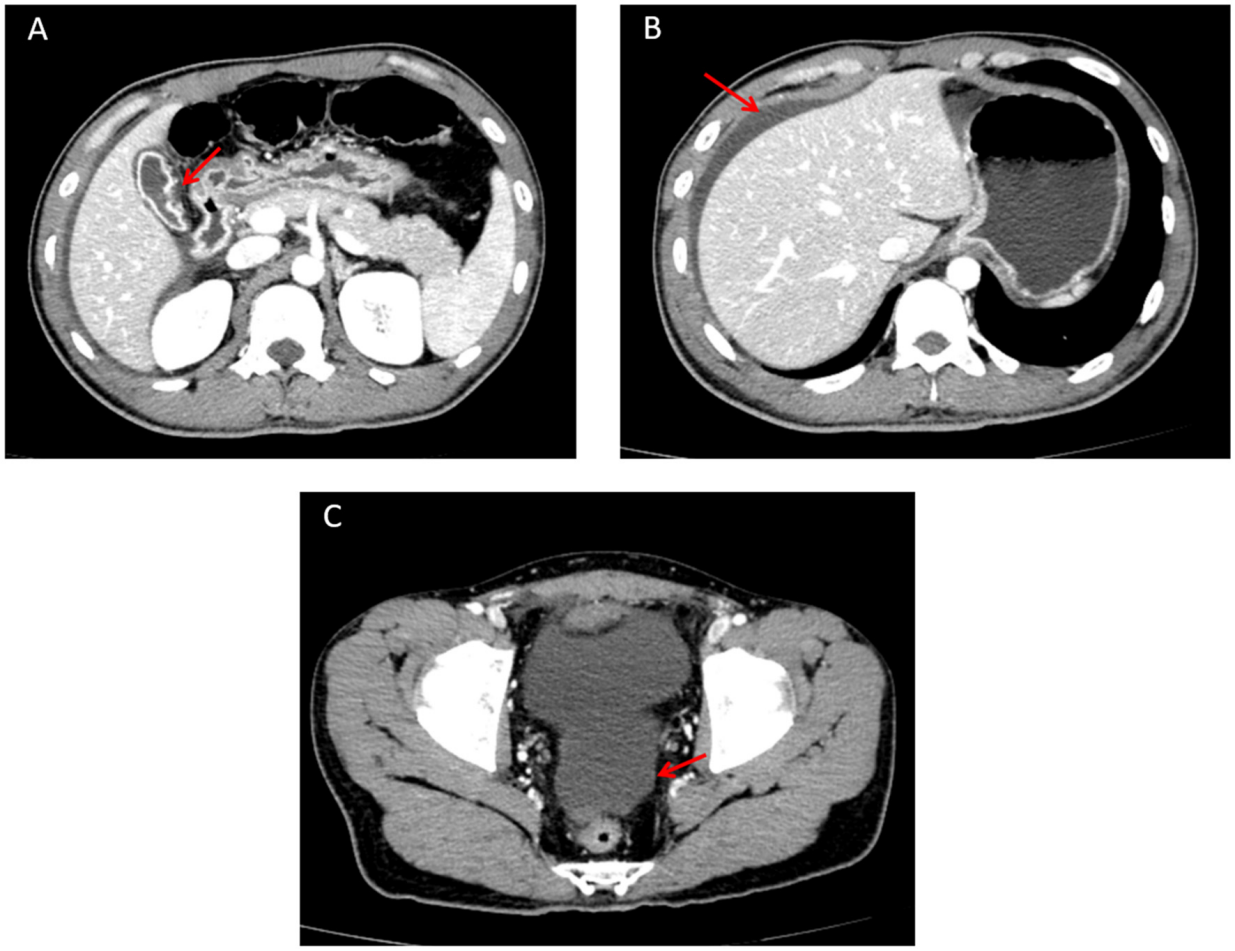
Abdominal contrast-enhanced CT image. (A) Perforated gallbladder (showing interrupted continuity and indistinct margins of the gallbladder wall adjacent to the duodenum) on abdominal computed tomography. (B) Perihepatic fluid collection, with the liver demonstrating a smooth outer margin, normal morphology, size, and hepatic lobe proportions, on abdominal computed tomography. (C) Pelvic fluid collection on abdominal computed tomography.
